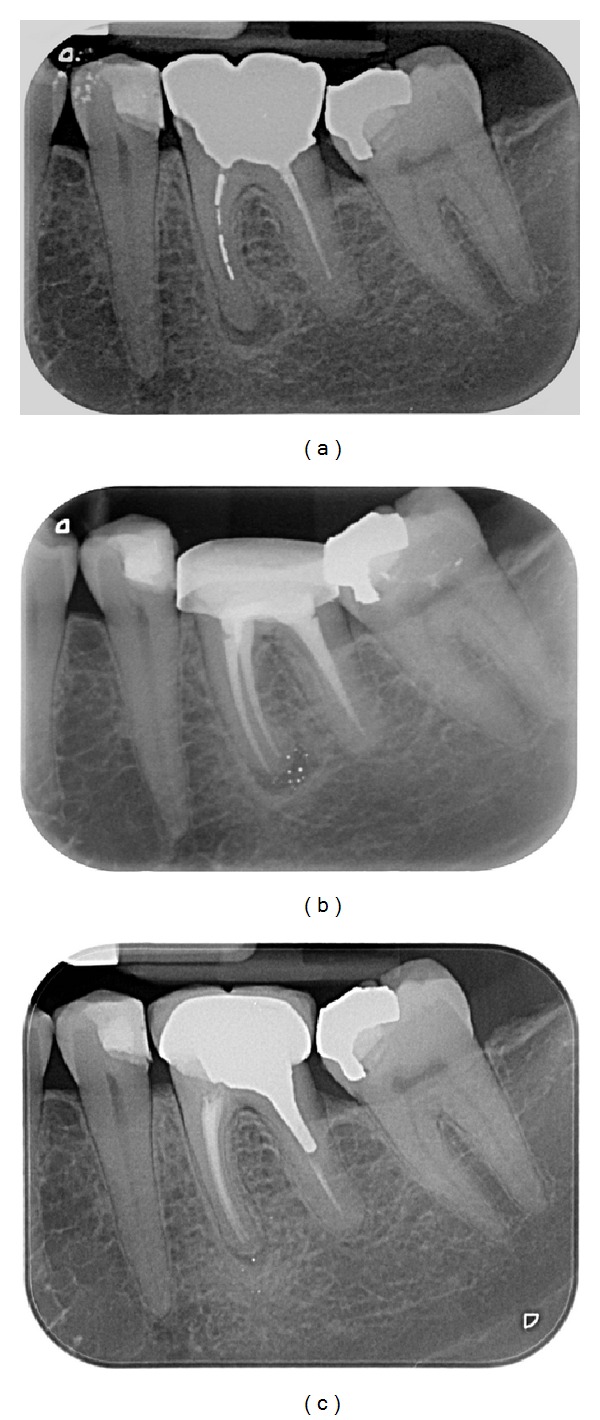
Case classified as healing. (a) Preoperative X-ray of the 36. Patient referred for orthograde retreatment of 36, asymptomatic, with an apical chronic periodontitis on the mesial root. Note the apical radiolucency (PAI = 3) and pieces of amalgam in the mesial root. The initial endodontic treatment was performed more than one year ago. (b) Periapical X-ray control immediately after the retreatment: amalgam grains are visible in the periapical region after realisation of the endodontic retreatment. (c) Recall after 4 years: this tooth is classified as “healed”: the amalgam grains seem to have disappeared but there is still a radiolucent area (PAI = 2).
